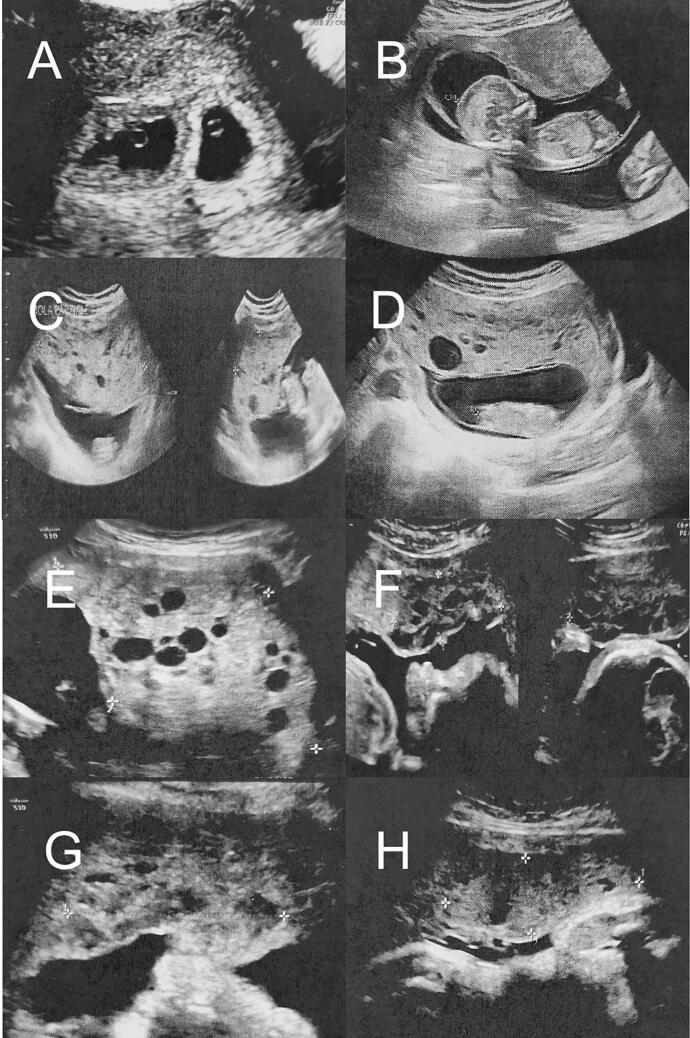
Ultrasound findings per gestational age. A Dichorial-diamniotic pregnancy (5 + 5 weeks of pregnancy). B Living fetus (13 + 1 weeks of pregnancy) with normal placenta. C Honeycomb like placental mass 10.4 × 4.5 × 4.7 cm (13 + 1 weeks of pregnancy). D Honeycomb like placental mass 10 × 10 × 3.5 cm (15 + 4 weeks of pregnancy). E Honeycomb like placental mass 11.2 × 6.6 cm (17 + 2 weeks of pregnancy). F Honeycomb like placental mass 6.8 × 5.9 × 2.5 cm (25 + 1 weeks of pregnancy). G Honeycomb like placental mass 8.2 × 5.7 × 2.2 cm (31 weeks of pregnancy). H Honeycomb like placental mass 7.8 × 7.9 × 2.6 cm (37 weeks of pregnancy).
